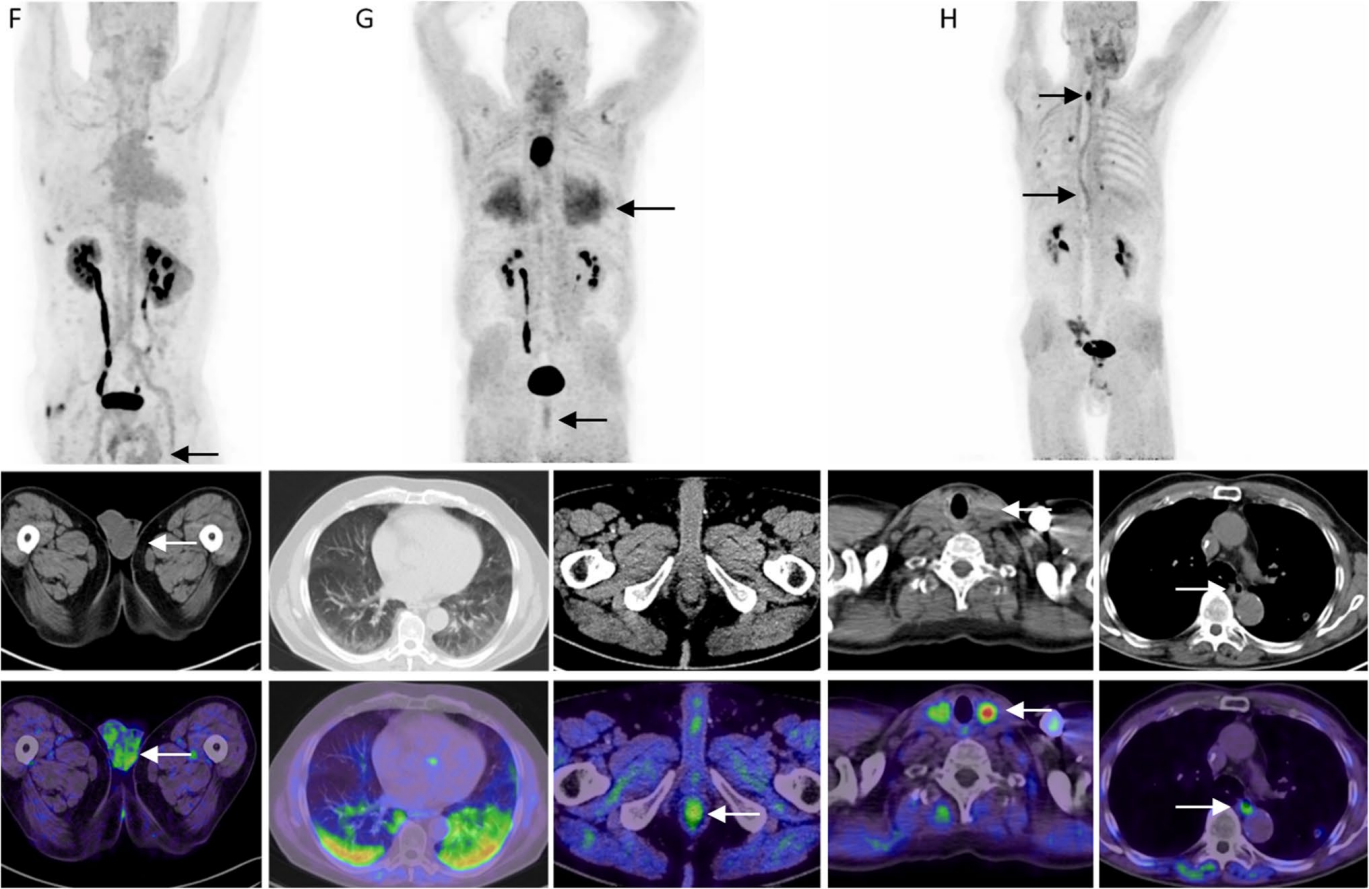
F A 60-year-old man with renal cell carcinoma showed a moderate, diffuse uptake of the testicles. As further side findings, BPH was noted. G A 76-year-old patient with esophageal cancer showing a diffuse, bilateral, high uptake of FAPI in the lungs, predominantly in the inferior lobe, fibrosis of the lung was diagnosed in the CT scan. Furthermore, this patient also showed moderate, diffuse uptake in the anal canal. No discernible pathologies were noted in the rectum or anal canal. H A 68-year-old-patient with colon cancer and two incidental findings; an intense uptake in the left lobe of the thyroid was noted. TSH levels were normal, and scintigraphy was recommended. Furthermore, the patient showed a moderate uptake in the middle and lower third of the esophagus without discernible esophageal pathologies in the CT scan or medical history.
